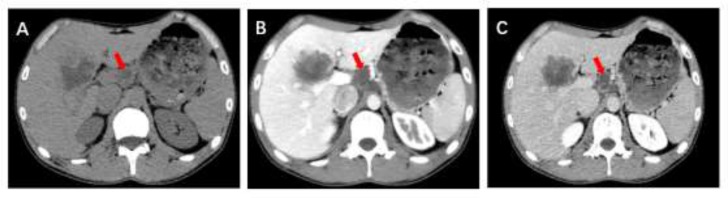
CT plain and enhanced scan. (A) Hydatid lesion in liver and enlarged lymph node in the hepatogastric space with diameter of 2.13 cm. B (portal vein phase) and C (delayed phase) showed circular enhancement.
